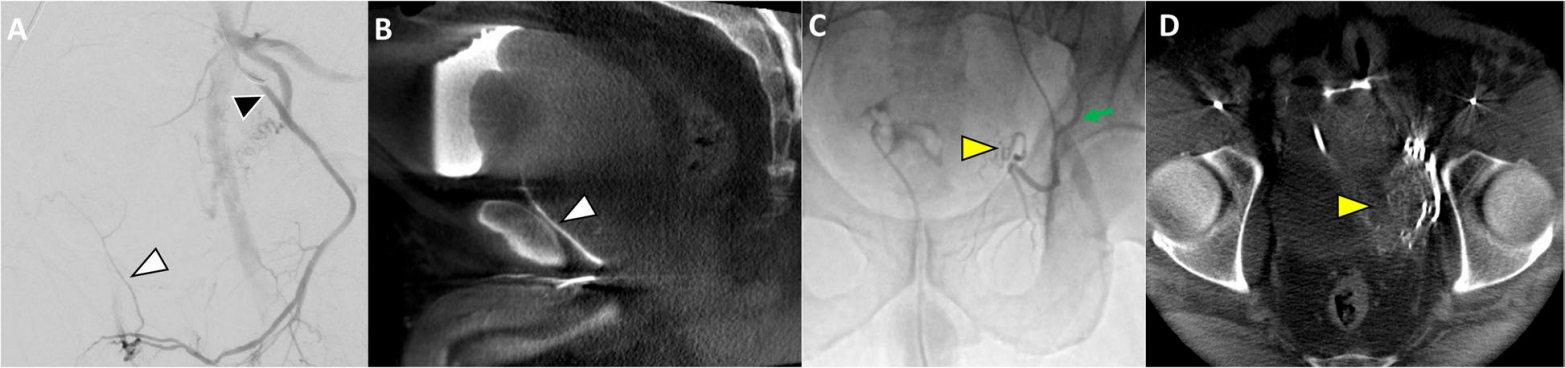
A Selective DSA of the left internal pudendal artery (black arrowhead) did not reveal a definite prostatic artery or obturator artery. A terminal branch of the left internal pudendal artery is seen extending cephalad (white arrowhead). B Sagittal reconstruction of a contrast enhanced CBCT with selective injection in this terminal branch (white arrowhead) demonstrated perfusion of the median lobe. C&D) evaluation of the left inferior epigastric artery was then performed (green arrow), which confirmed that the prostatic artery (yellow arrowhead) was arising from the aberrant left obturator artery (corona mortis). Interestingly, this patient also had a right prostate artery origin from an aberrant right obturator artery (not shown)
